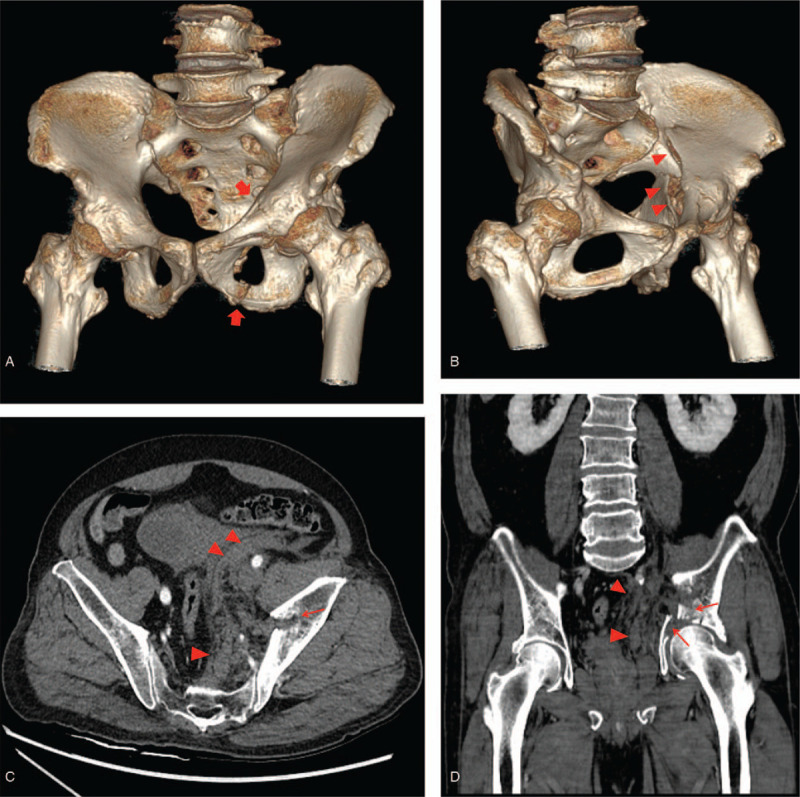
A 64-year-old man was admitted to our trauma center after falling from a height of 2 m. (A and B) A volume-rendered 3D-reconstructed image shows multiple fractures involving the left superior and inferior rami (red arrow) and a comminuted left iliac bone fracture (red arrow head). The suggested fracture type is the Young and Burgess Classification of lateral compression type II. (C and D) Axial and coronal CT scans demonstrate a comminuted left iliac fracture (thin red arrow) with a pelvic side wall hematoma (red arrow head); no evidence of trauma-related vascular injury is observed.
